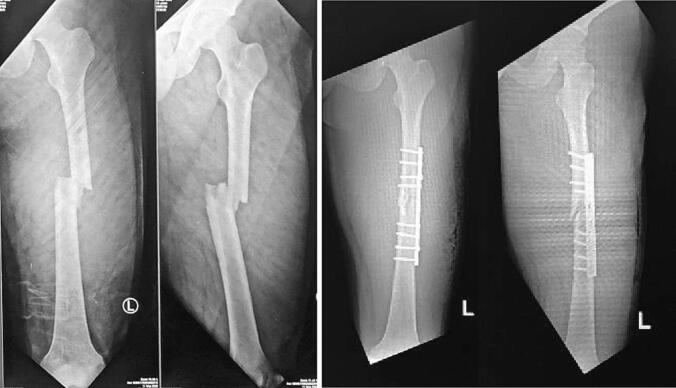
Initial presentation to emergency department and post-operative x-ray with plate fixation.
